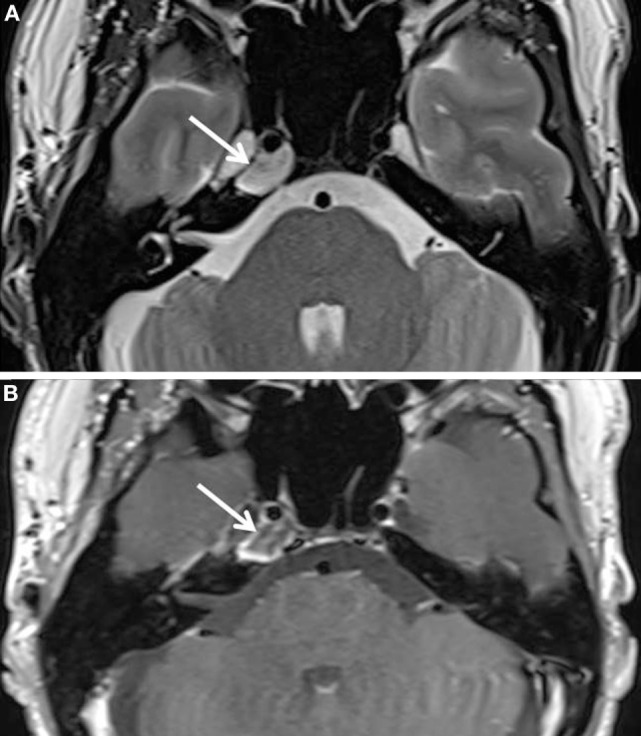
Postoperative MRI images. (A) T2-weighted axial and (B) T1-weighted axial images after injection of gadolinium (B) show a notable regression of the right petrous apex lesion (white arrows). The content of the lesion is still heterogeneous. There is slight peripheral enhancement.
